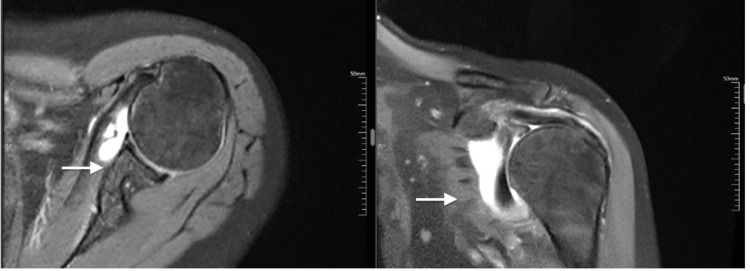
MRI (axial and coronal sections) showing supraspinatus tendon tear with acromioclavicular joint arthropathy and joint effusion (proton density/short TI inversion recovery sequences, white arrows, white arrows)
